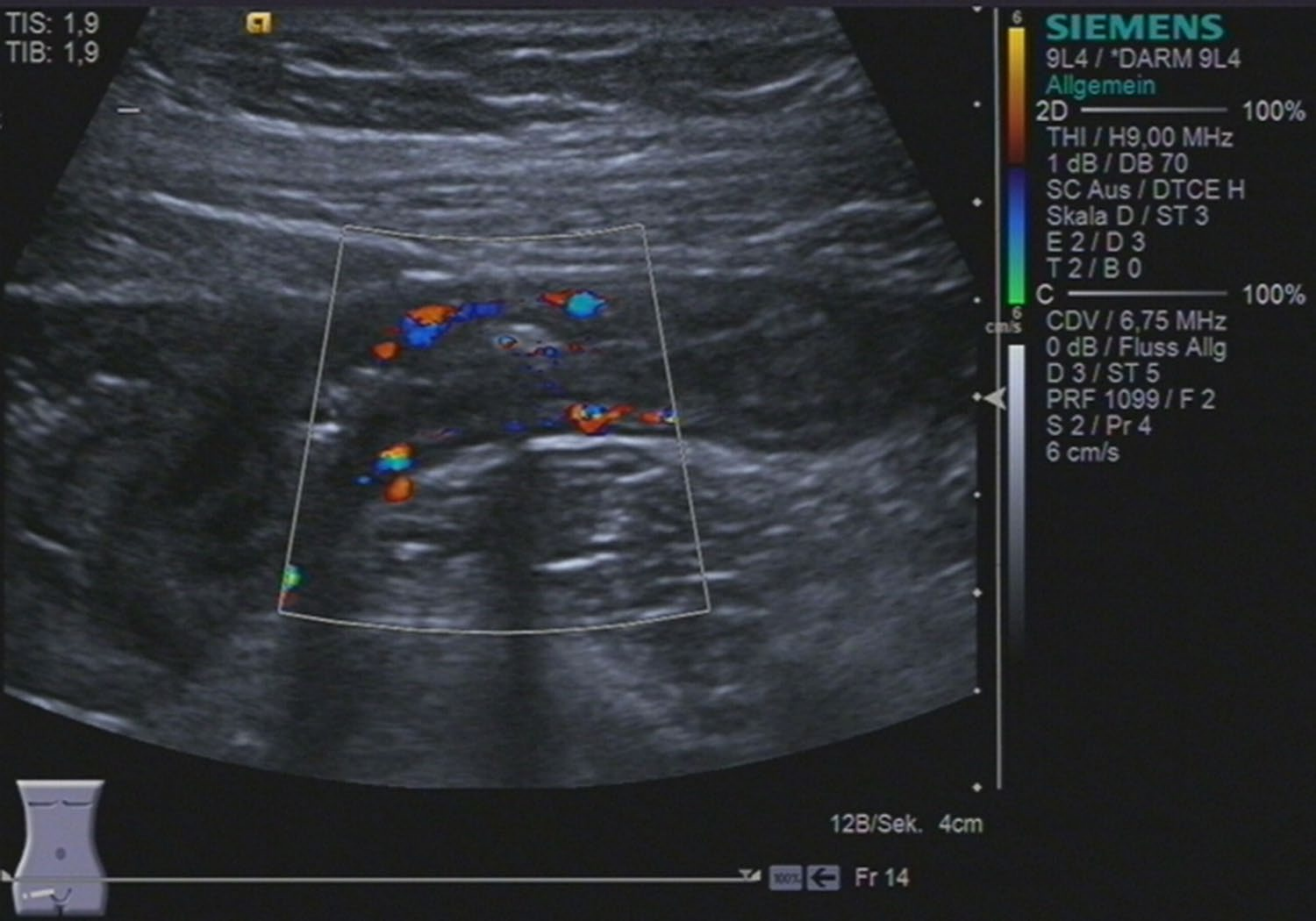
Sonographically active Crohn’s ileitis with 3.6 mm bowel wall thickening and a Limberg score of 3
